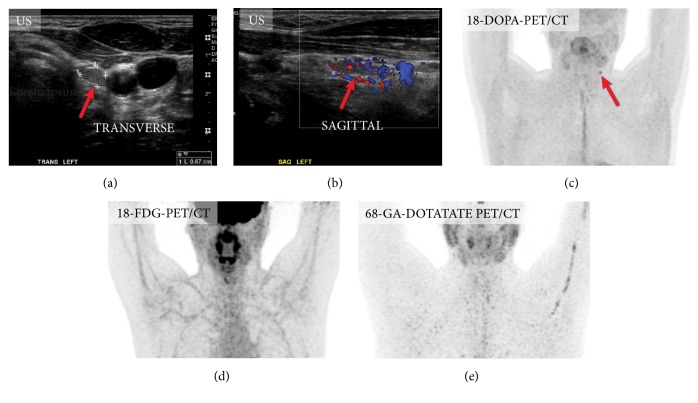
Mapping of the metastatic lymph nodes in medullary thyroid carcinoma. Asymptomatic patient after thyroidectomy for medullary thyroid carcinoma and elevated calcitonin level (54 pg/ml) was evaluated for possible neck lymph node metastases. Ultrasound of the neck showed several suspicious lymph nodes (not shown). One of the lymph nodes (b) was found to be positive on only 18F-FDOPA-PET/CT (c), while 18F-FDG-PET/CT and 68Ga-DOTATATE-PET/CT were negative.
